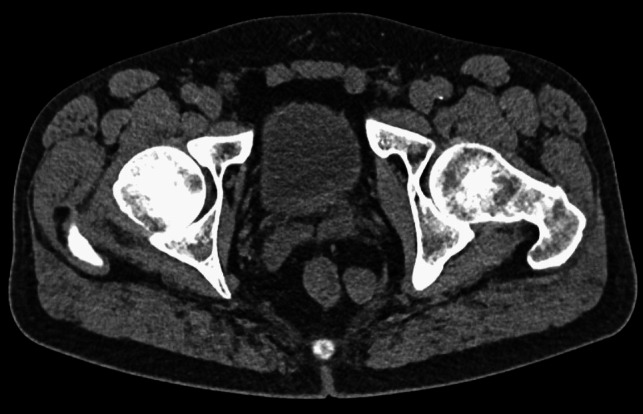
Pelvic CT scan showing a 2.3 × 1.9 × 2.0 cm soft tissue mass within the left mesorectal fascia
